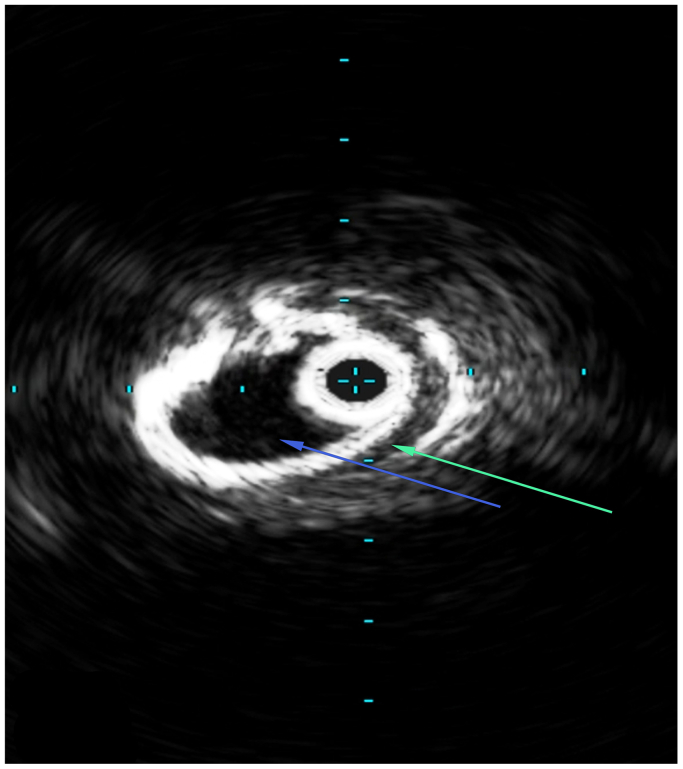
Intravenous ultrasound (IVUS) depicting the cadaveric aorta prior to repair. The *blue arrow* indicates the true lumen and the *green arrow* indicates the false lumen.
